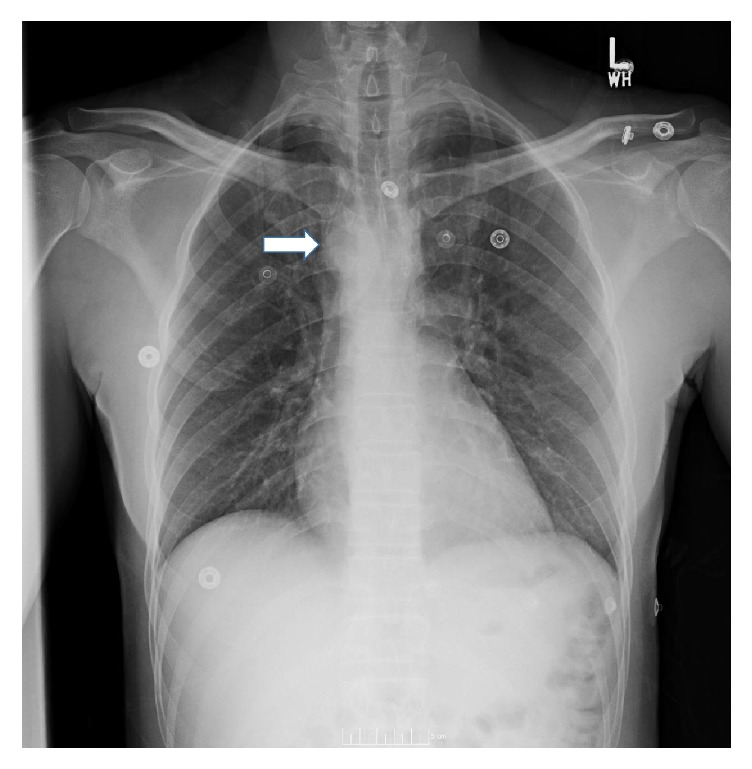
Radiograph of the chest posteroanterior view, with the right sided aortic arch (white arrow).
